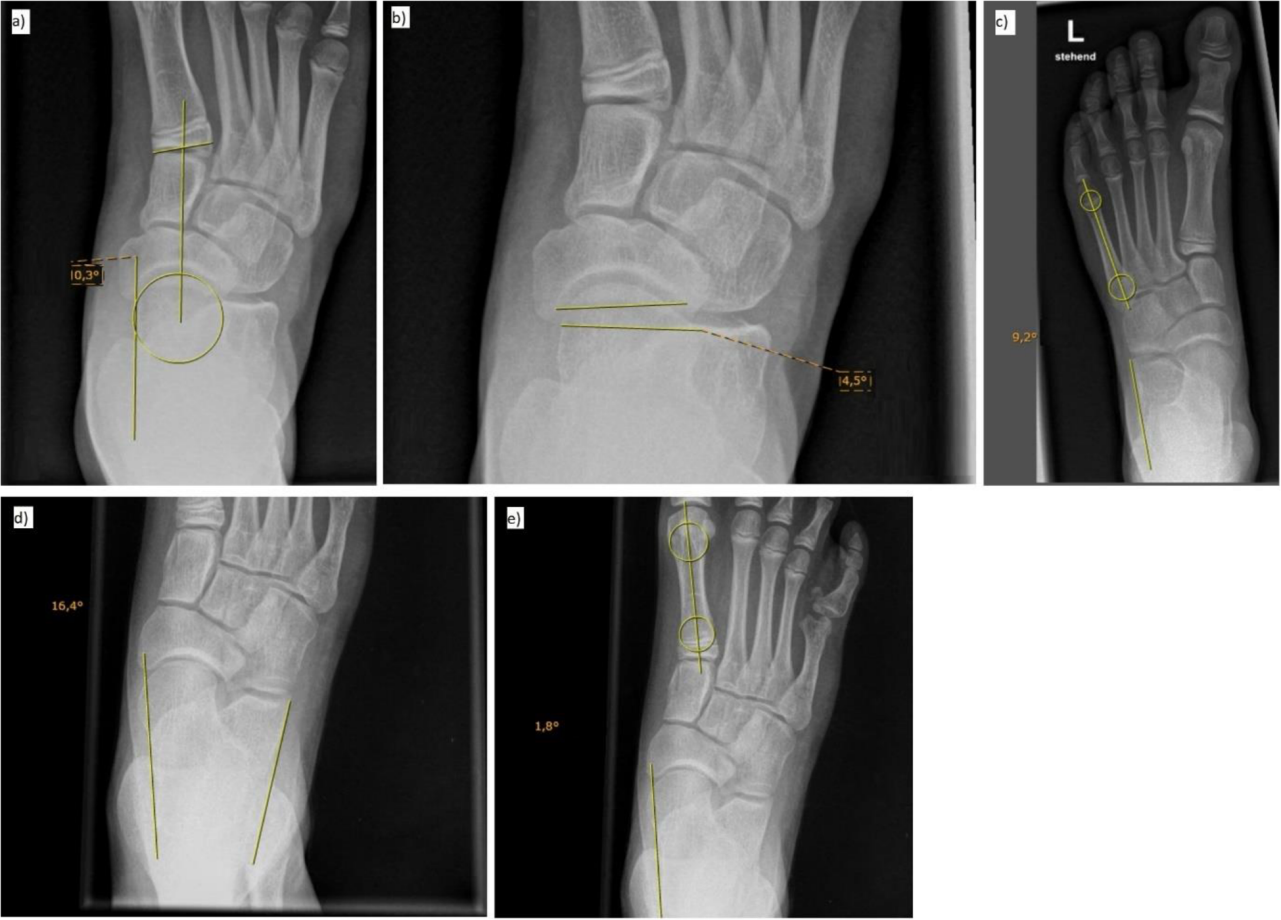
Radiographic parameters evaluated in the dorsoplantar view: a Talo-first-metatarsal-base angle (TMTIB), (b) Talonavicular coverage (TNC), (c) Calcaneus-fifth-metatarsal angle (Calc-MTV), (d) Talocalcaneal angle (TC-dp), (e) Talo-first-metatarsal angle (TMTI-dp)
